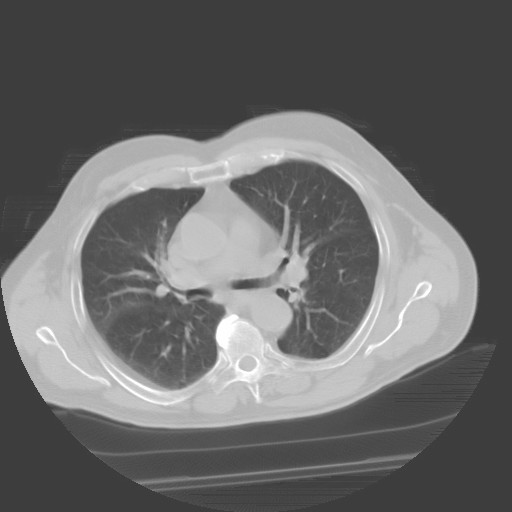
CT scan of the chest showing right lower lobe scarring and left upper lobe small nodules with focal pleural thickening.
